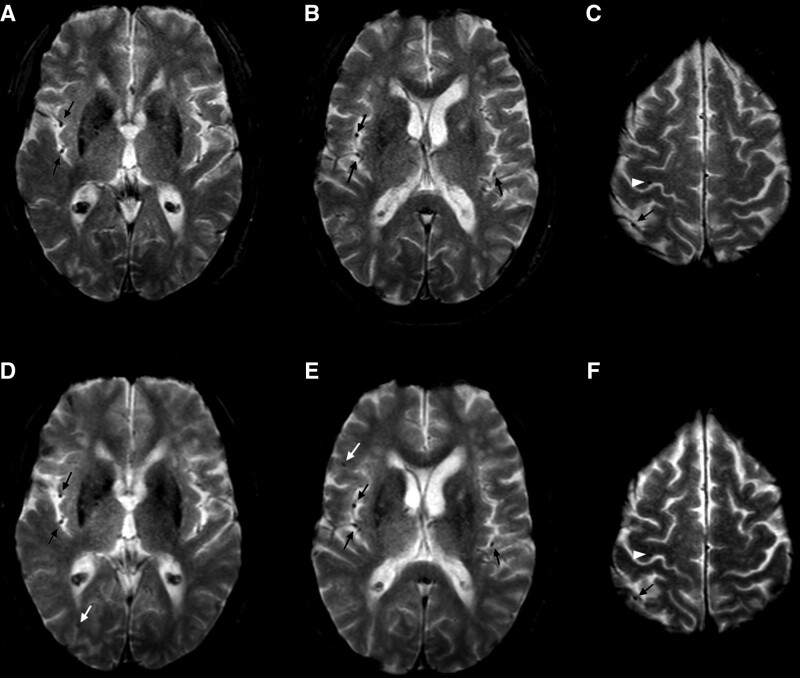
T2*-weighted gradient-echo sequence before and after transcatheter aortic valve implantation. Before (A–C) and after (D–F) transcatheter aortic valve implantation. This patient had a unique microbleed in the right precentral gyrus before transcatheter aortic valve implantation (white arrowhead; C). Two new microbleeds were observed after transcatheter aortic valve implantation, in the right frontal and temporal lobes (white arrows; D and E). Cerebral microbleeds were identified as homogeneous, round foci, <10 mm diameter, of low signal intensity. Flow void artifacts of the pial blood vessels (black arrows) were clearly distinguished from cerebral microbleeds by their location in the subarachnoid space and their tubular morphology on the adjacent slices. Preoperative and postoperative T2* images have been coregistered with an automated coregistration software dedicated to longitudinal comparison of magnetic resonance images using rigid transformations on the basis of mutual information (Longitudinal Brain Imaging; Philips Medical Systems).
